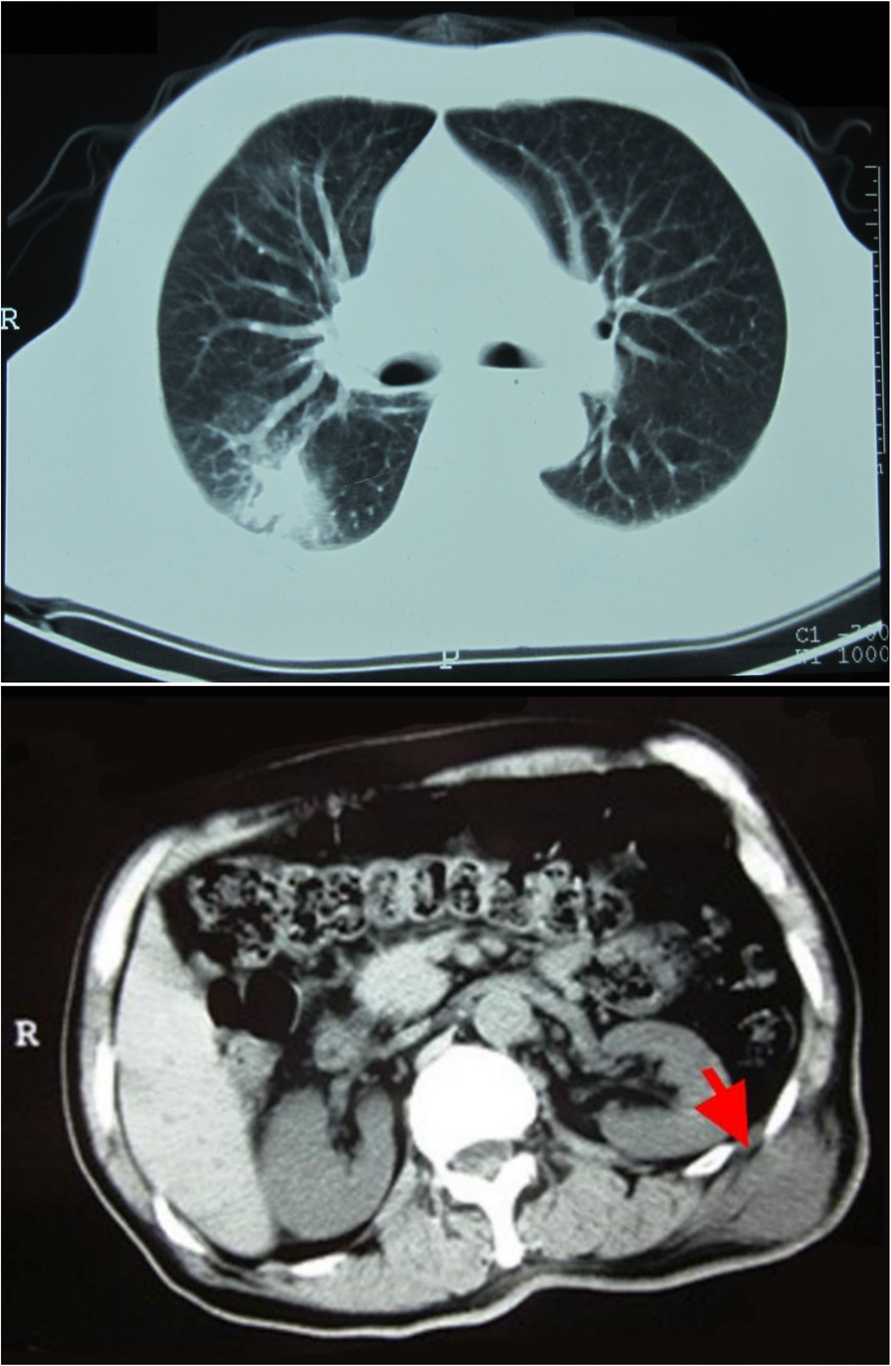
Abscess in lung and fascia: Chest CT scan revealed pulmonary abscess formation (*above*) and an abscess inside the fascia (*below*)
